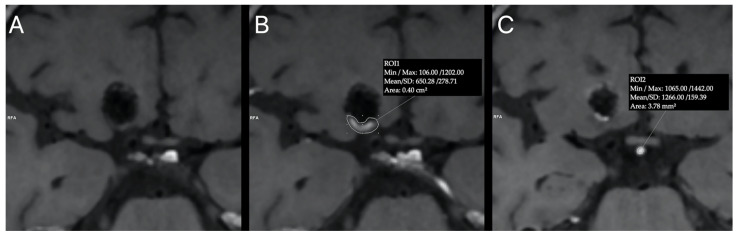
Example of region of interest (ROI) placement for quantitative analysis of post-procedural aneurysm contrast enhancement intensity. Precontrast (A) and postcontrast (B,C) HR-VW MRI of right internal carotid artery aneurysm treated with stent-assisted coiling. ROI drawn manually around the area of peripheral aneurysm contrast enhancement (B). Second ROI positioned within the pituitary stalk (C).
